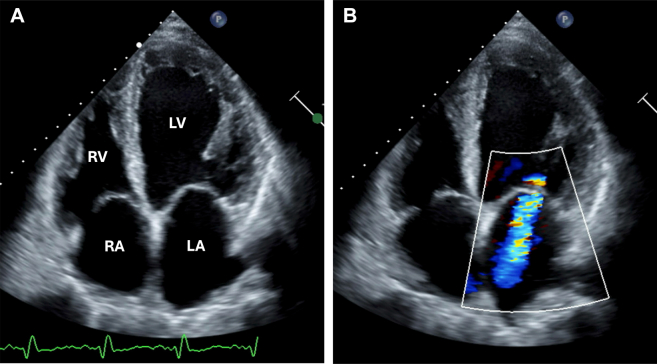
Transthoracic Echocardiography Findings (A) Transthoracic echocardiography showed severe dilation of the left ventricle (LV), with severely reduced LV systolic function (ejection fraction: 20%). The right ventricle (RV) was mildly dilated with mildly reduced function. The left atrium (LA) and right atrium (RA) were dilated. (B) There was moderate mitral and tricuspid regurgitation with color Doppler shown over the mitral valve.
